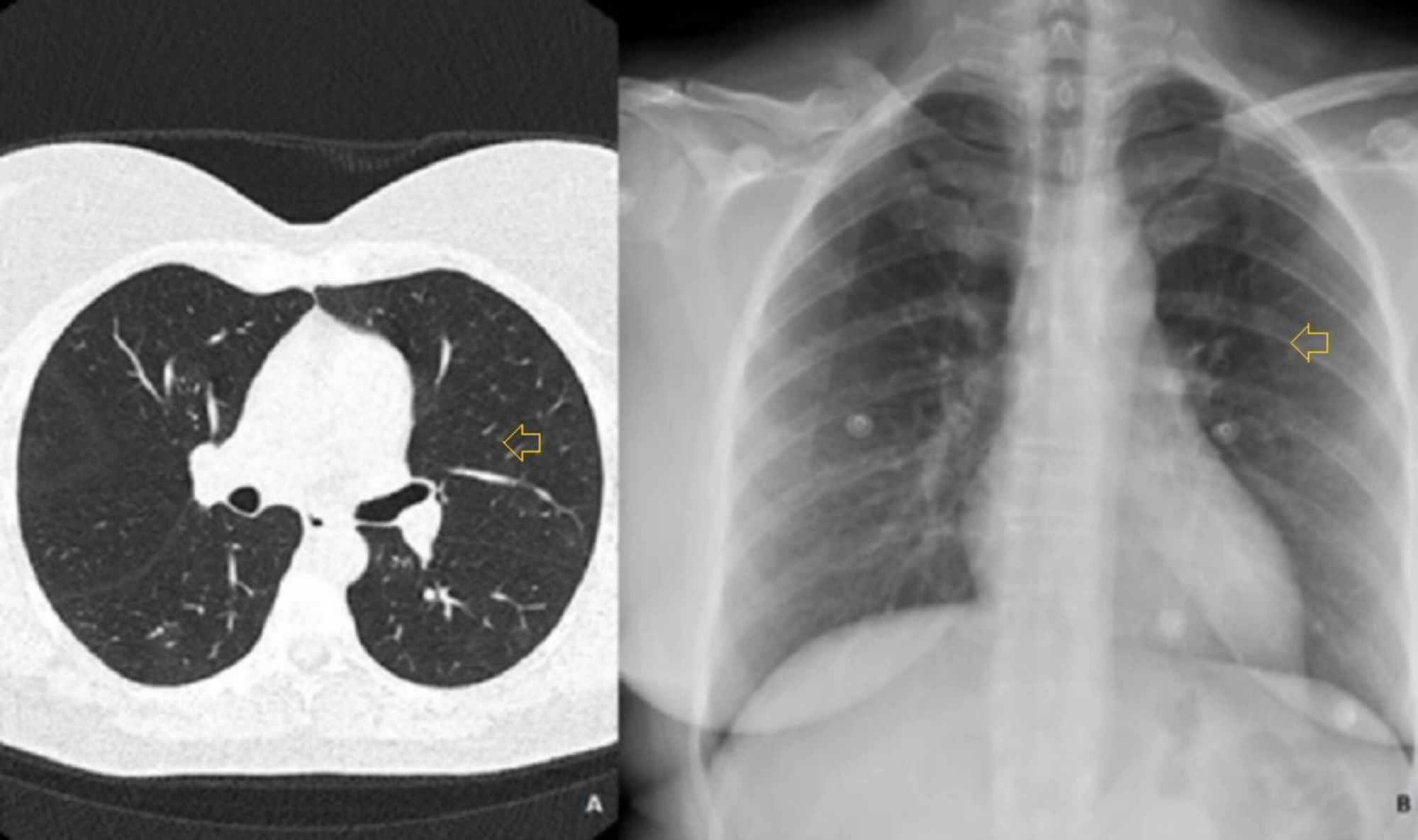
a. CT chest scan, b. Posteroanterior chest radiography No thoracic abnormalities were noted.
